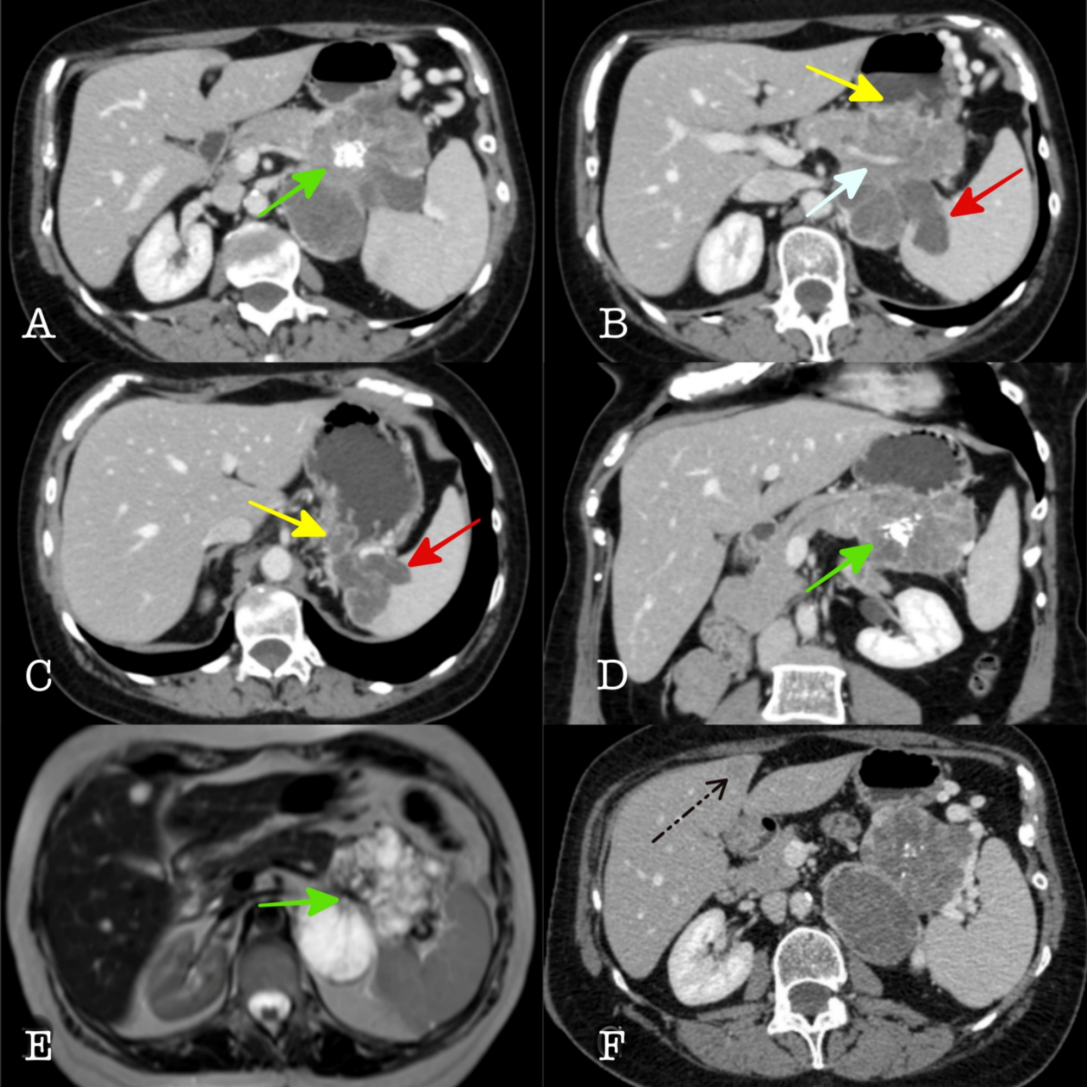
(A,B,C,F) Contrast enhanced axial CT slices, D) Oblique coronal CT reconstruction, and E) T2 axial MRI show a large lobulated, multicystic mass with central calcified scar. Multicystic appearance and central calcified scar (green arrows) resemble the imaging features of the more common benign serous cystadenoma (microcystic adenoma), however, invasion of splenic vessels (white arrow), spleen (red arrows), stomach wall (yellow arrows), and liver metastases (black arrow) indicate a more aggressive, malignant lesion.
